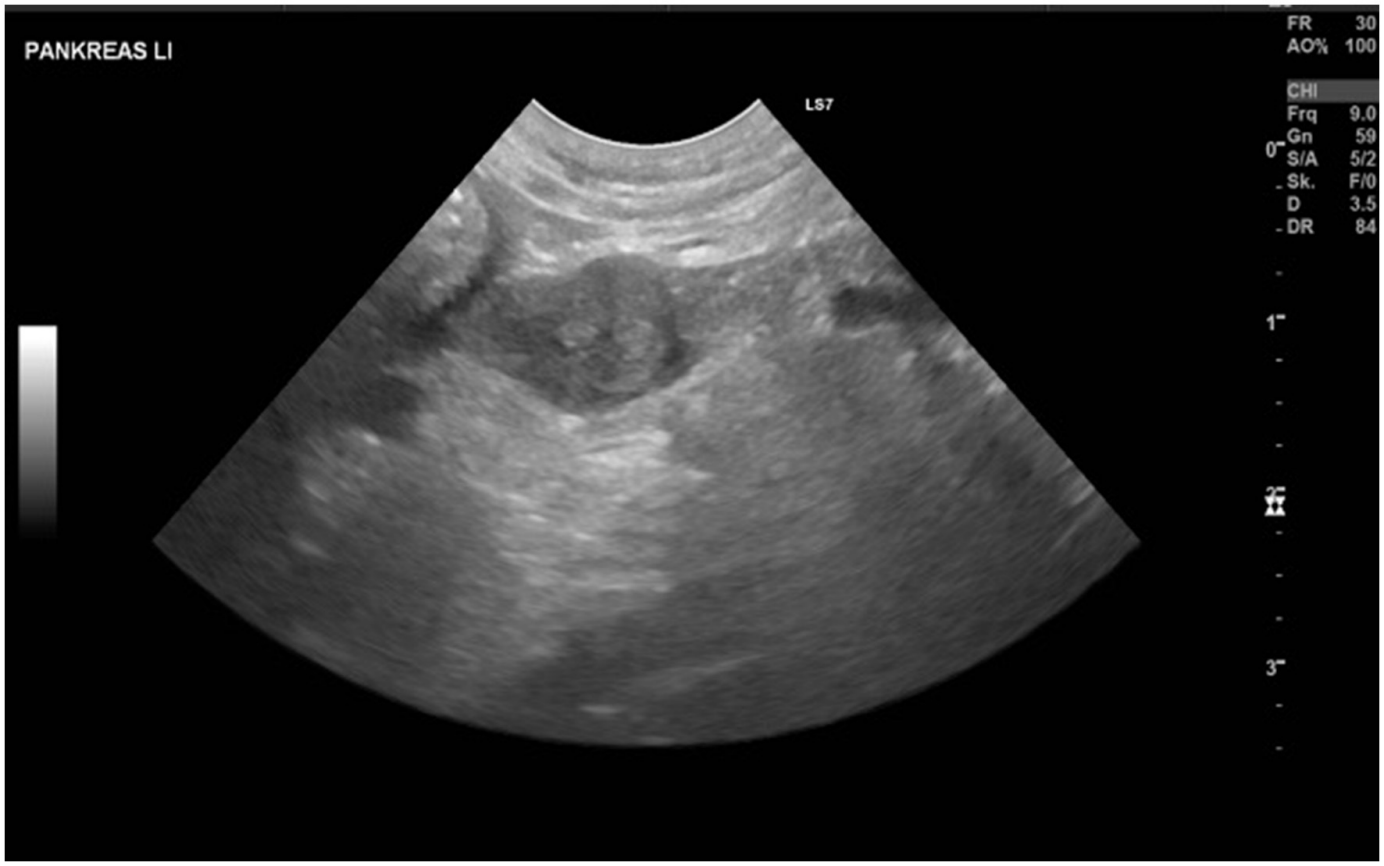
Showing the lesion as well as the enlarged lymph node detected at the initial ultrasound examination.
